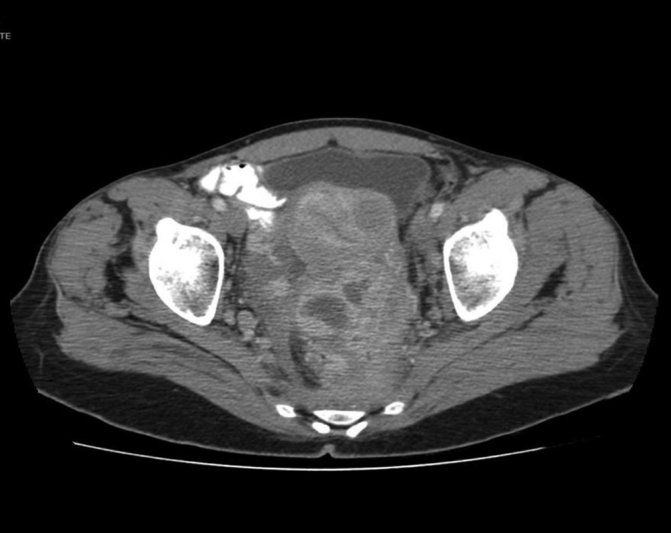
Axial abdominopelvic computed tomography scan image showing a large heterogeneous pelvic mass.
